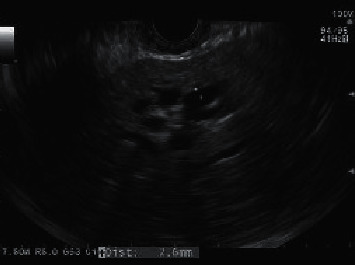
Endoscopic ultrasound showing a dilating segment 3 biliary radicle.
